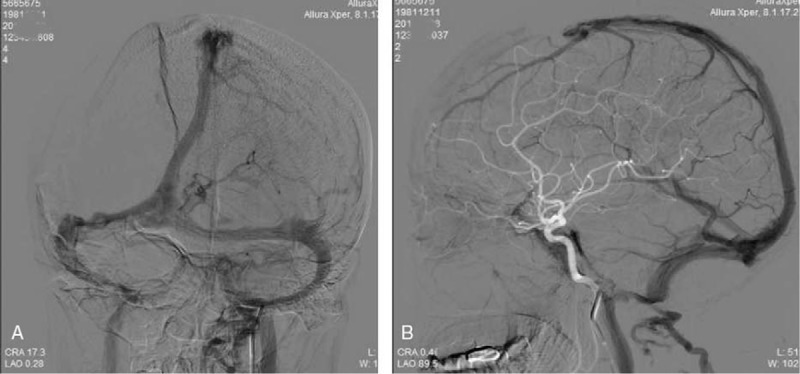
The results of DSA of 3 months after endovascular treatment. A. Posteroanterior radiograph; B. Lateral imagine. DSA = digital subtraction angiography.
